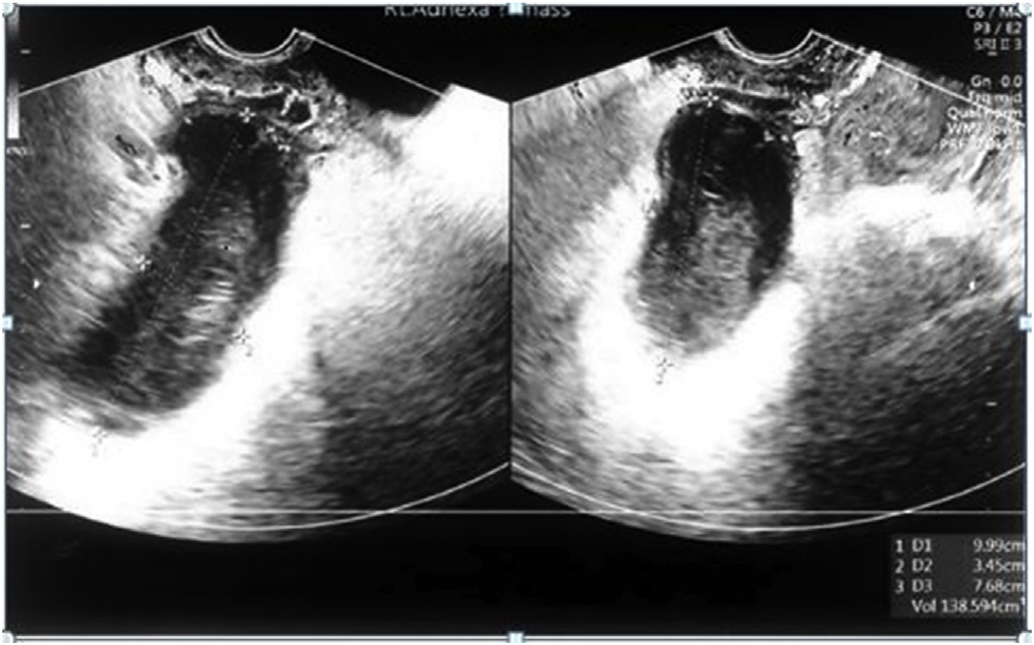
Ultrasound showing an elongated hypo-echoic thick-walled tubule-cystic mass measuring 9.9 x 3.4 × 7.7cm with complex heterogeneous with internal echoes in the right adnexa.
